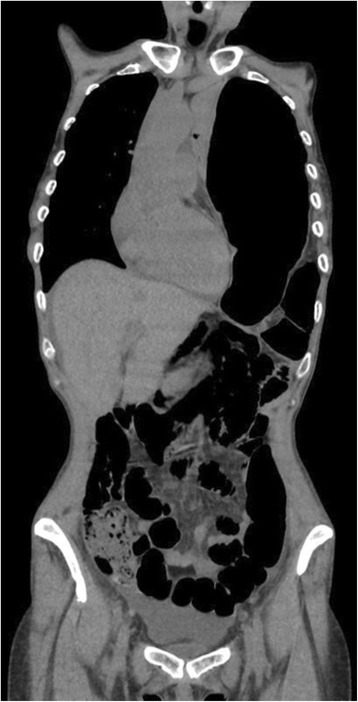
CT scan shows in *left side*, large diaphragmatic defect which allows passage of the stomach, spleen and colon (referred to as *Bochdalek* hernias) and complete collapse of left lung
